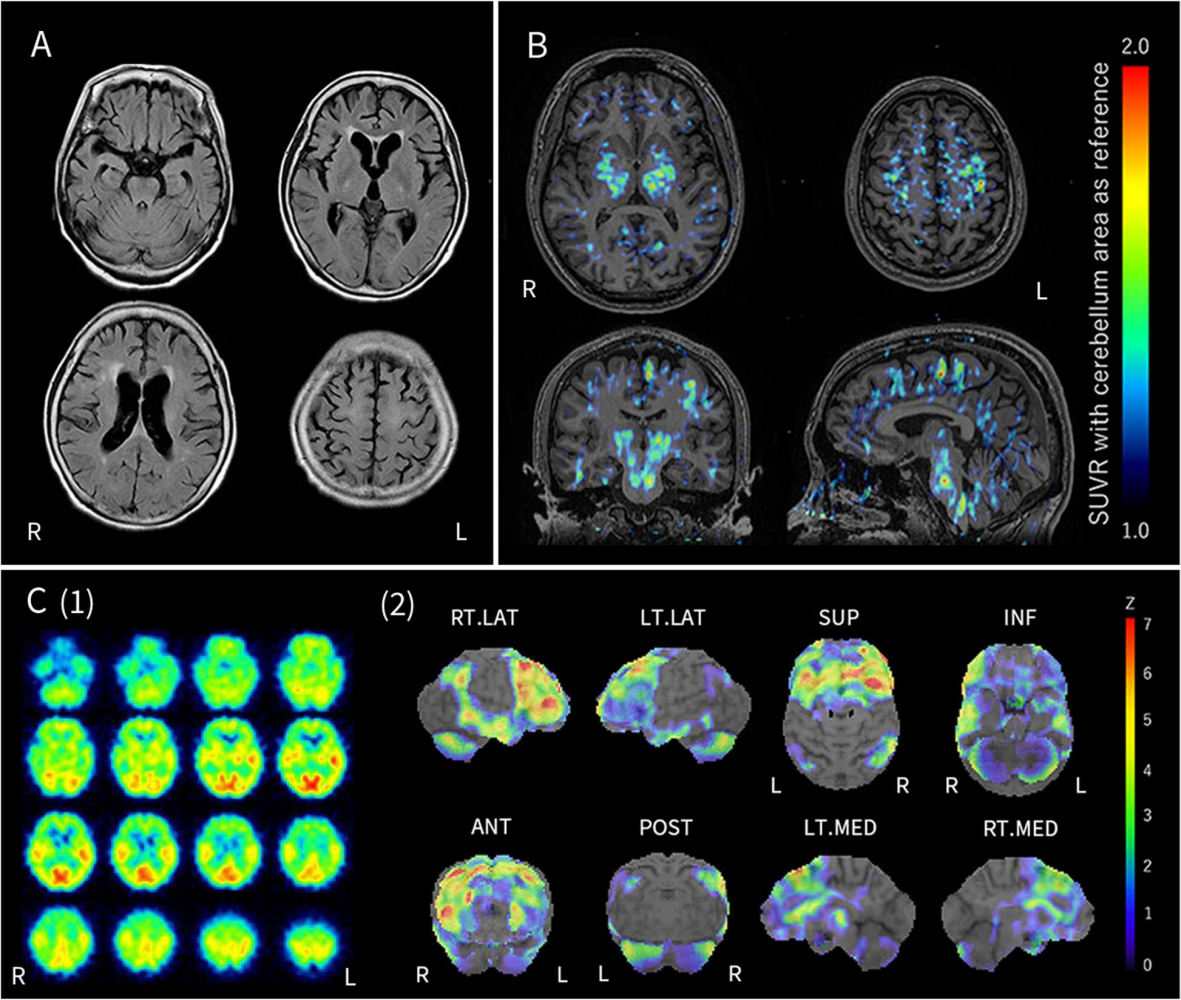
MRI, tau PET, and perfusion SPECT images. Brain magnetic resonance imaging revealed bilateral frontotemporal lobar atrophy on T2/fluid-attenuated inversion recovery (FLAIR) (A). Tau positron emission tomography (PET) with Florzolotau (^18^F) demonstrated remarkable uptake of the ligand around the brainstem, subthalamic nucleus, basal ganglia, and bilateral subcortical frontal lobe (B). ^123^I-N-isopropyl-p-iodoamphetamine (^123^I-IMP) single-photon emission computed tomography (SPECT) showed reduced cerebral blood flow in the bilateral frontal lobe and basal ganglia (C-1). Easy Z-score imaging system (eZIS) analysis of ^123^I-IMP SPECT imaging also revealed significantly decreased regional cerebral blood flow (rCBF) in bilateral frontal areas, including the anterior operculum (C-2). SUVR, standardized uptake value ratio; RT, right; LT, left; LAT, lateral; SUP, superior; INF, inferior; ANT, anterior; POST, posterior; MED, medial
